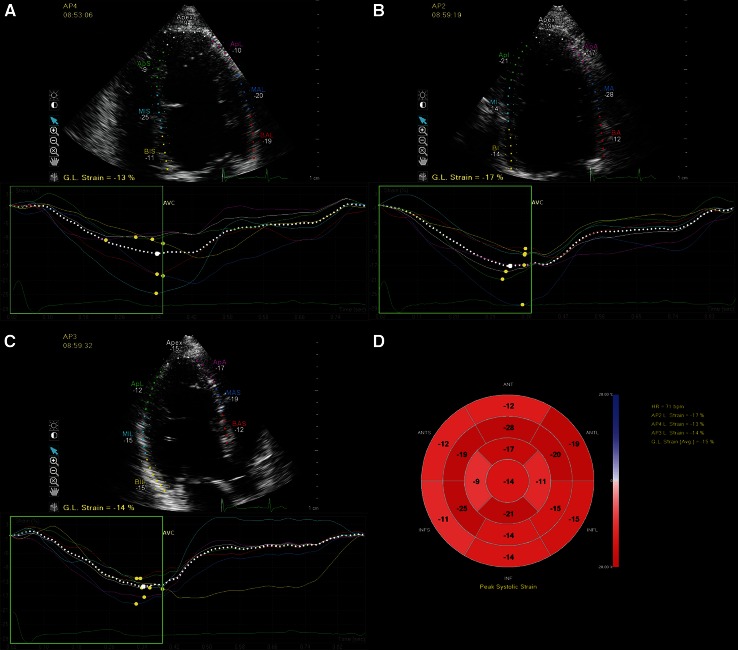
Example of left ventricular longitudinal strain measurements in a patient with repaired aortic coarctation. The LV was traced in the apical four-, two-, and three-chamber views at end-diastole. The walls were automatically divided into seven segments at each view and the global longitudinal strain at each view was calculated (a–c). The segmental strain measurements were plotted in a bull’s eye and the left ventricular global longitudinal strain based on all three apical views was calculated (d). *AP2* apical two-chamber view, *AP3* apical three-chamber view, *AP4* apical four-chamber view, *GL* global longitudinal
